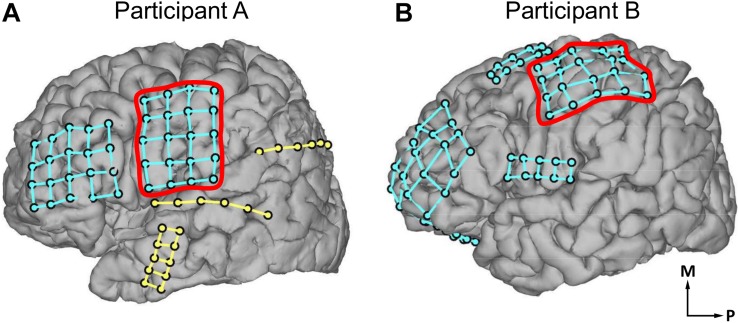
Locations of electrocorticogram electrodes on participants’ cortical surfaces as determined by coregistration of MRI and CT images. Electrode locations are visualized with dots, with different electrode grids distinguished by the presence of grid lines between those dots. (A) Electrode locations in participant A. (B) Electrode locations in participant B. In (A,B), red outlines indicate the electrode grids analyzed in the current study. The outlined electrode grid was on the left hemisphere of the brain for participant A, and the right hemisphere for participant B; participant B’s data has been mirrored throughout this report for comparison. Axes labels indicate medial (M) and posterior (P) directions.
